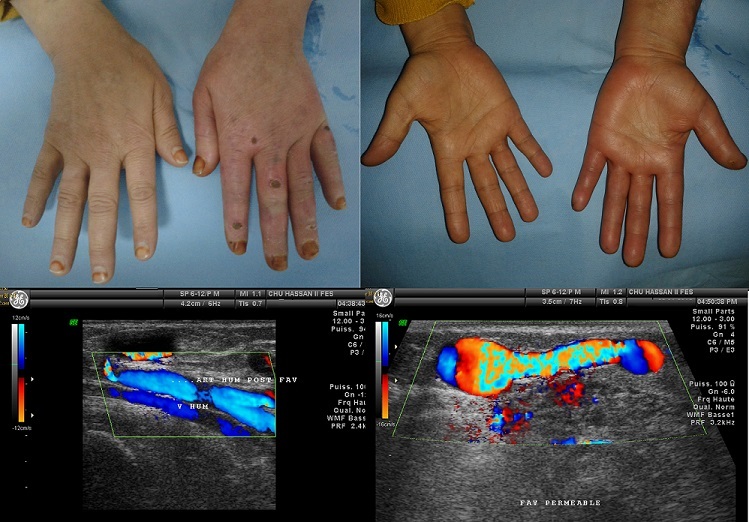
Aspect clinique de la main gauche présentant une coloration un peu bleutée avec des ulcérations digitales nécrotiques; le doppler couleur objective l’inversion de flux au niveau de l’artère humérale en aval de la fistule
